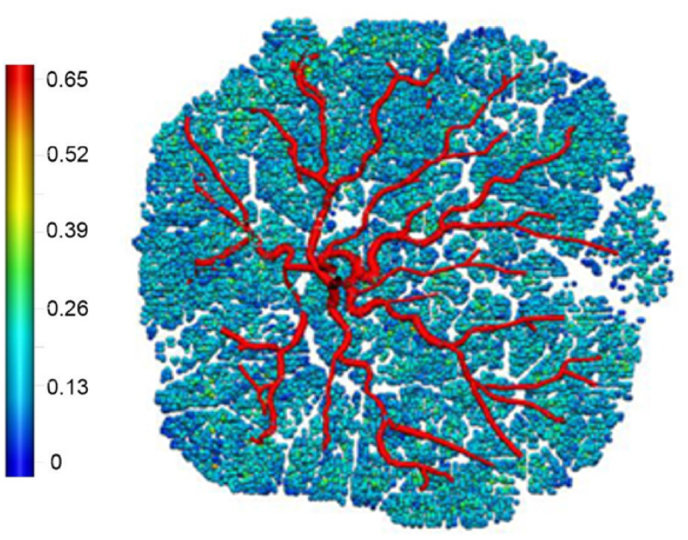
The multiscale flow model of an ex vivo human placenta from Micro CT quantifying the perfusion. The colour scale shows the volumetric flow in mm3/s reproduced with permission from Byrne et al. Approximate diameter of 20 cm [1].
